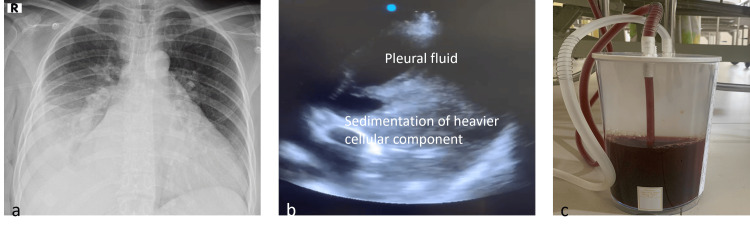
Chest radiography suggestive of right moderate pleural effusion (a), Ultrasound of the chest demonstrating the hematocrit sign, seen as fluid layering caused by sedimentation of red blood cells, suggestive of hemothorax. (b), Spictra showing haemorrhagic drain from the intercostal drainage tube (c).
